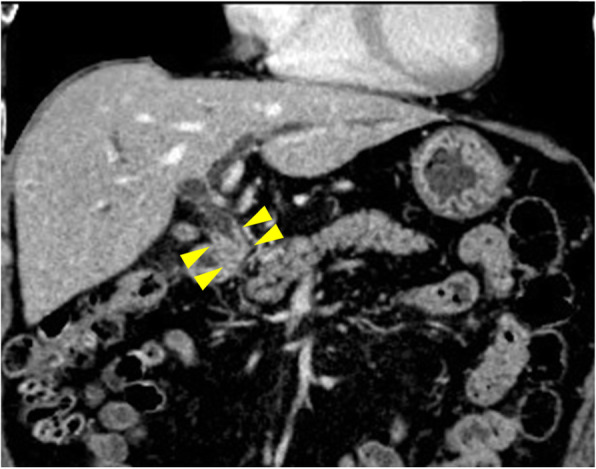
Enhanced wall thickening from the middle to the distal portion of the common bile duct (arrow) without any enlarged regional lymph nodes was detected by enhanced CT (coronal section image)
